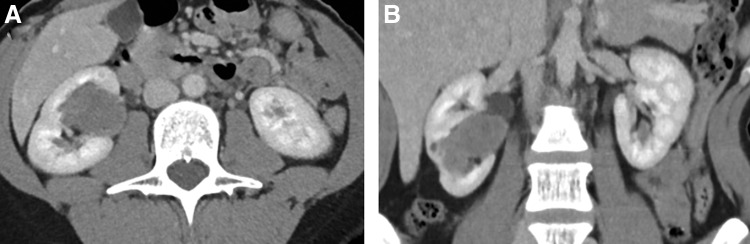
CT scan with axial (A) and coronal (B) views showing heterogeneously enhancing mass within the right kidney.
